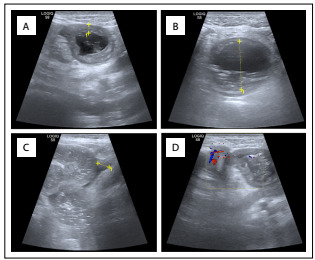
Abdominal ultrasonography: (A) A distended bowel can be seen below the umbilicus, and peristalsis is not apparent. The wall of the tube is thickened, and the lumen at the beginning of its expansion is compressed. (B) A dark area is seen between the intestines. (C) The intestinal wall is raised into the lumen, and the two intestinal tubes share the same wall of the bowel canal. (D) A small amount of colored blood flow signal can be seen on the wall of the dilated intestinal tube.
